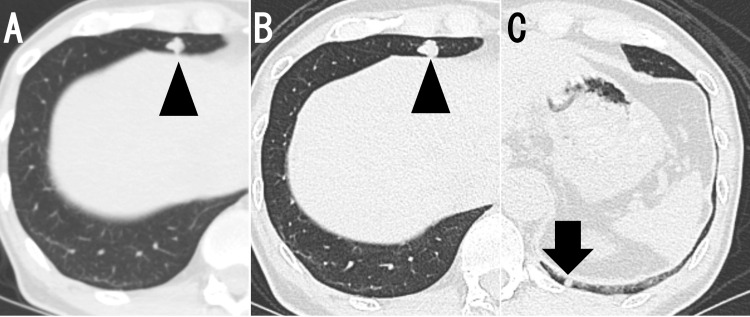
Chest CT A: Plain axial CT image showing a 10-mm nodule in the right middle lobe (arrowhead). B: The nodule enlarged to 12 mm four months later (arrowhead). C: Another nodule emerged in the left lower lobe (arrow).
